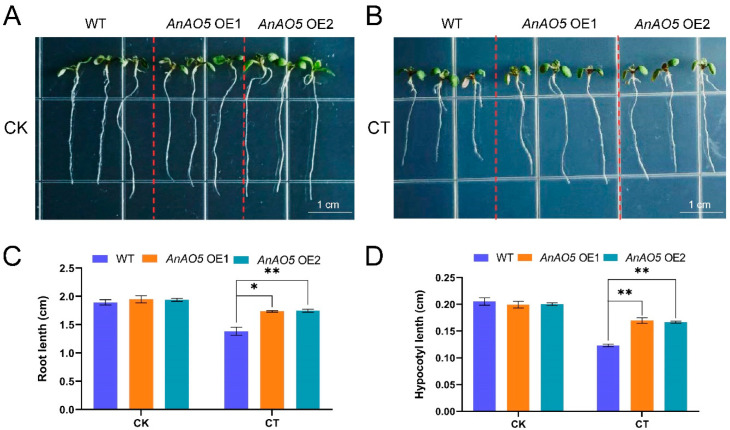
Overexpression of *AnAO5* enhanced the tolerance of Arabidopsis seedlings to low temperature. (A) Arabidopsis seedlings of wild-type, AnAO5 OE1, and AnAO5 OE2 cultured at room temperature (22 °C). (B) Arabidopsis seedlings of wild-type, AnAO5 OE1, and AnAO5 OE2 cultured under cold stress (15/10 °C). (C) The root lengths of Arabidopsis seedlings of wild-type, AnAO5 OE1, and AnAO5 OE2 in CK and CT. (D) The hypocotyl lengths of Arabidopsis seedlings of wild-type, AnAO5 OE1, and AnAO5 OE2 in CK and CT. Values are expressed as means ± SD (*n* = 3), and statistically significant differences were evaluated using Student’s t-test, * *p* < 0.05,  *p* < 0.01.
